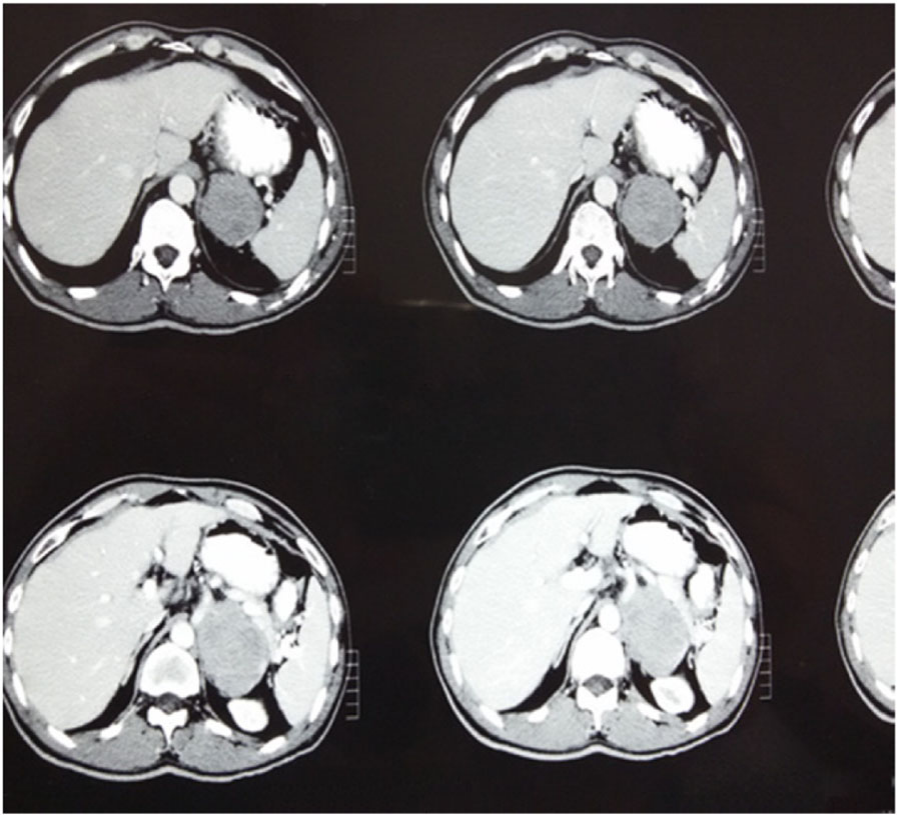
Computed tomography of the patient showing a large left adrenal mass
